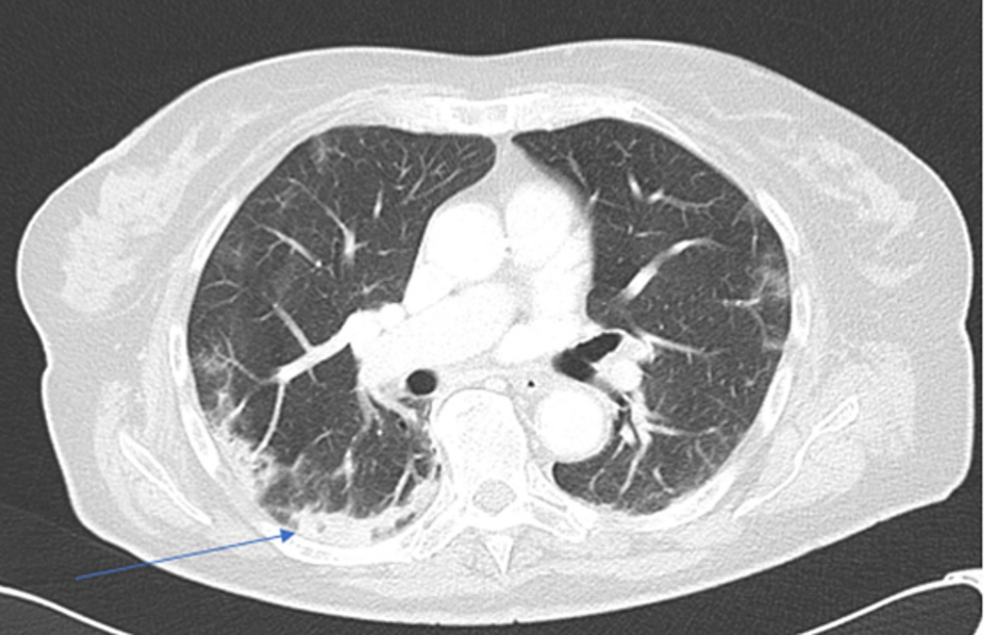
Thoracic CT showing areas of organizing pneumonia (arrow) CT, computed tomography
